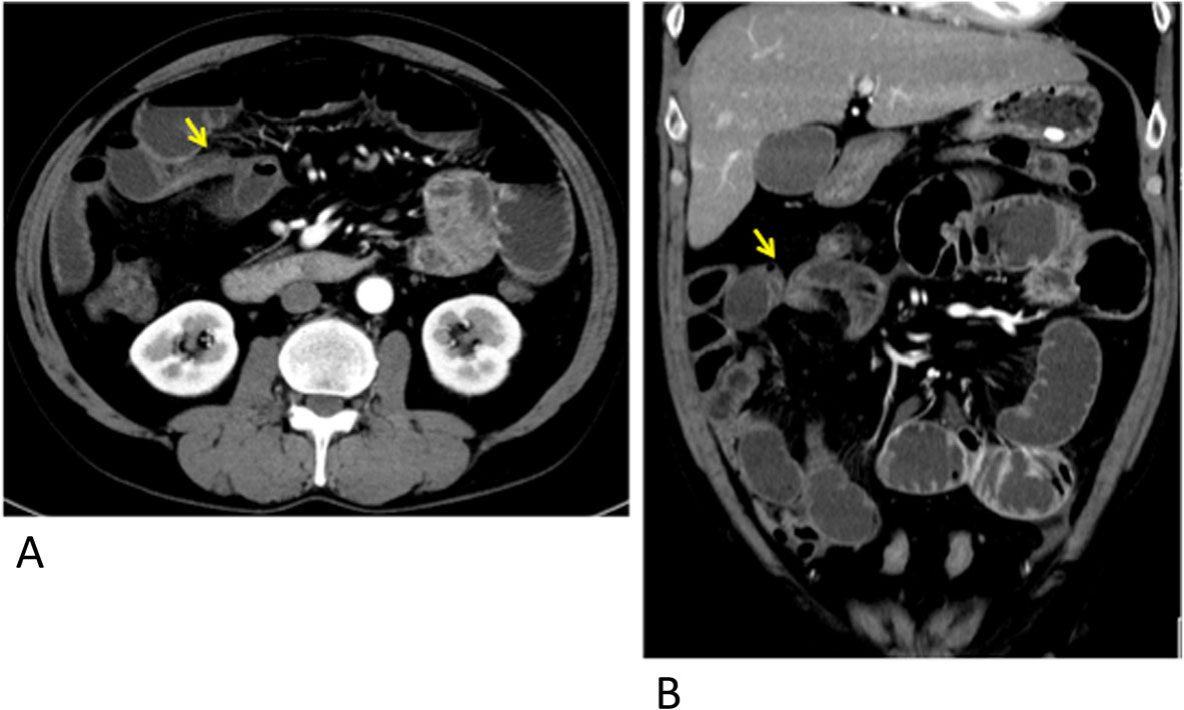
Enhanced abdominal computed tomography showing two caliber changes (arrows) that indicate a closed loop (a, b)
